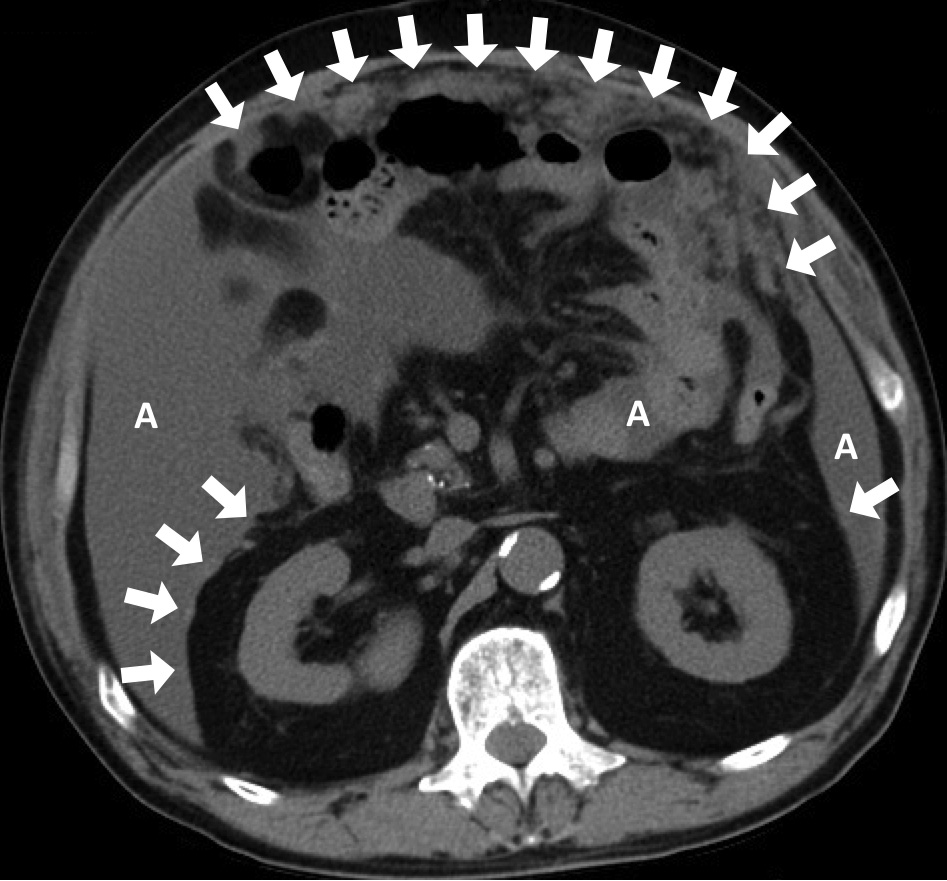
An abdominal CT scan shows mesenteric metastases (arrows) and ascites (A) due to peritoneal dissemination.
